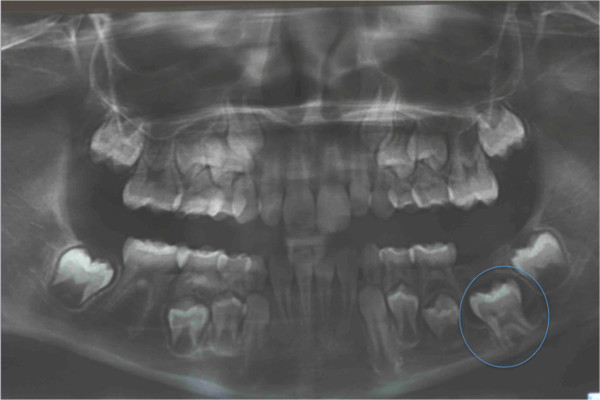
First orthopantomograph, before the patient came to our attention: a general dentist had suggested the extraction of the impacted left lower first molar (in the blue circle), after viewing this orthopantomograph.
